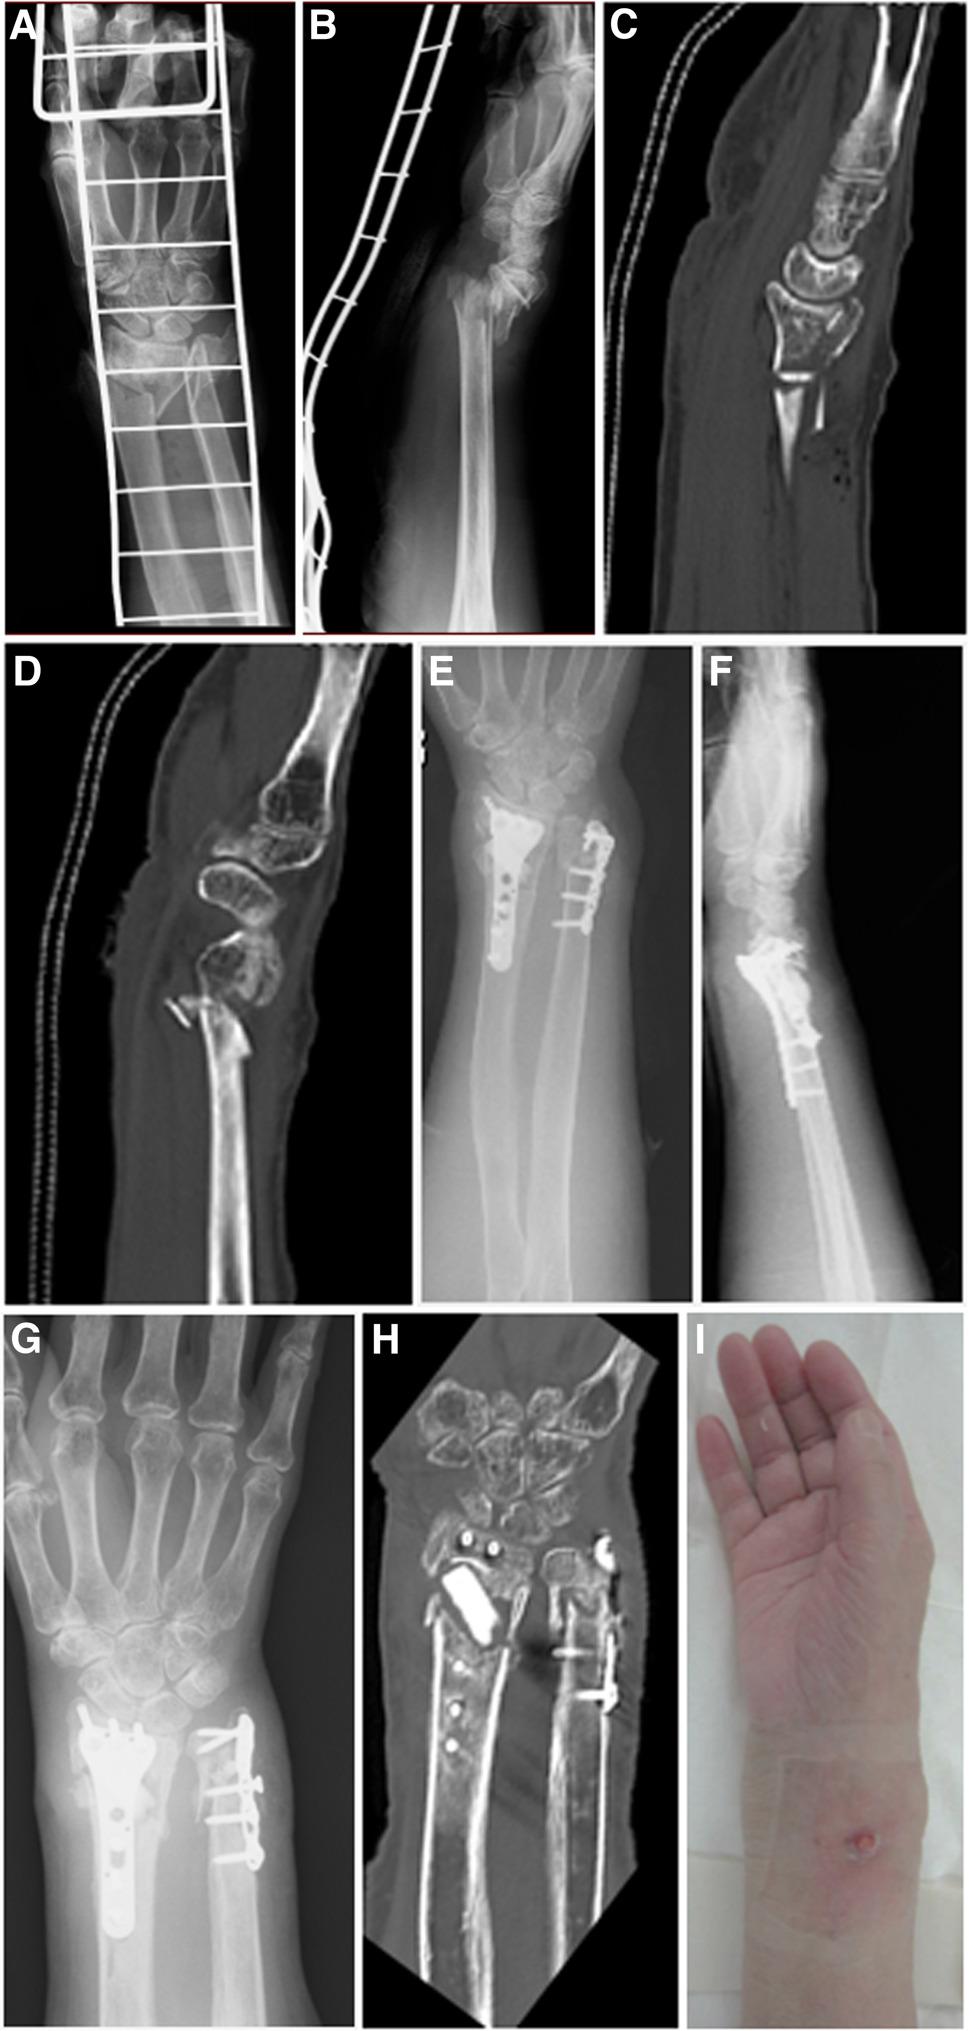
Clinical course from injury to referral. A Anteroposterior radiograph at the time of injury demonstrating an AO/OTA 23-C3 distal radius fracture. B Lateral radiograph at the time of injury showing metaphyseal comminution and displacement. C Immediate postoperative anteroposterior radiograph after volar plate fixation at the previous hospital. D Immediate postoperative lateral radiograph. E Three-month postoperative anteroposterior radiograph demonstrating persistent non-union. F Coronal CT image at three months showing incomplete osseous bridging and metaphyseal bone loss. G Clinical photograph at referral showing a draining sinus on the volar aspect of the wrist arising from the distal radial non-union site
